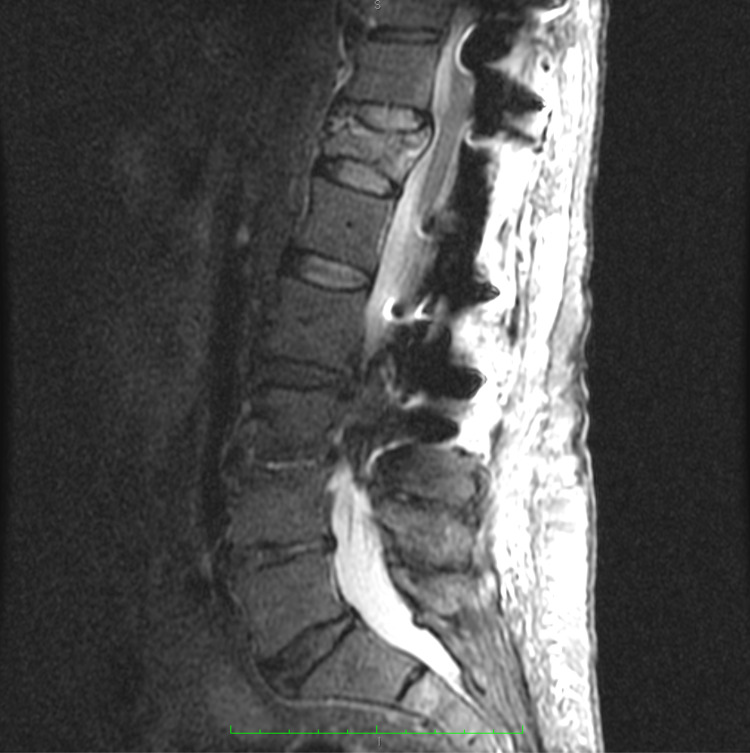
Preoperative STIR T2-weighted MRI Preoperative STIR T2-weighted MRI identifying acute to subacute burst fracture at T12 and artifact from the previously implanted hardware. STIR, short TI inversion recovery.
